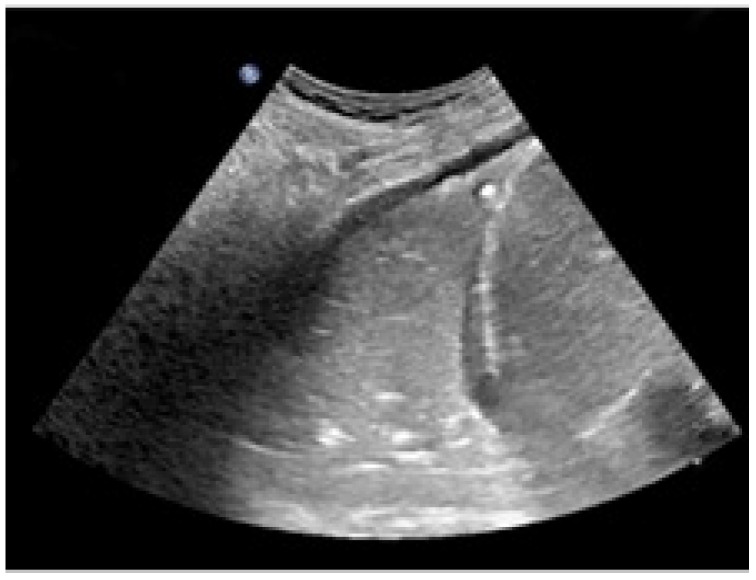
Left lung ultrasound showing a consolidation of the lung and the absence of the normal A lines.
